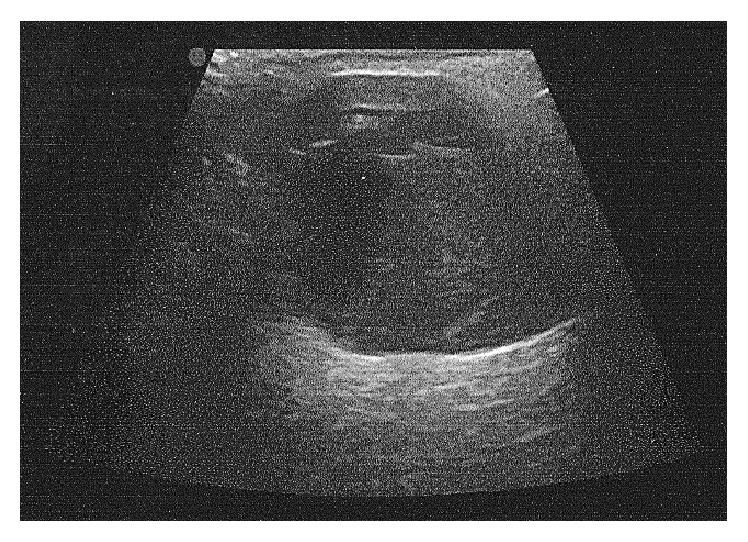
Scrotal ultrasonography with right ruptured implant and collapsed envelope.
